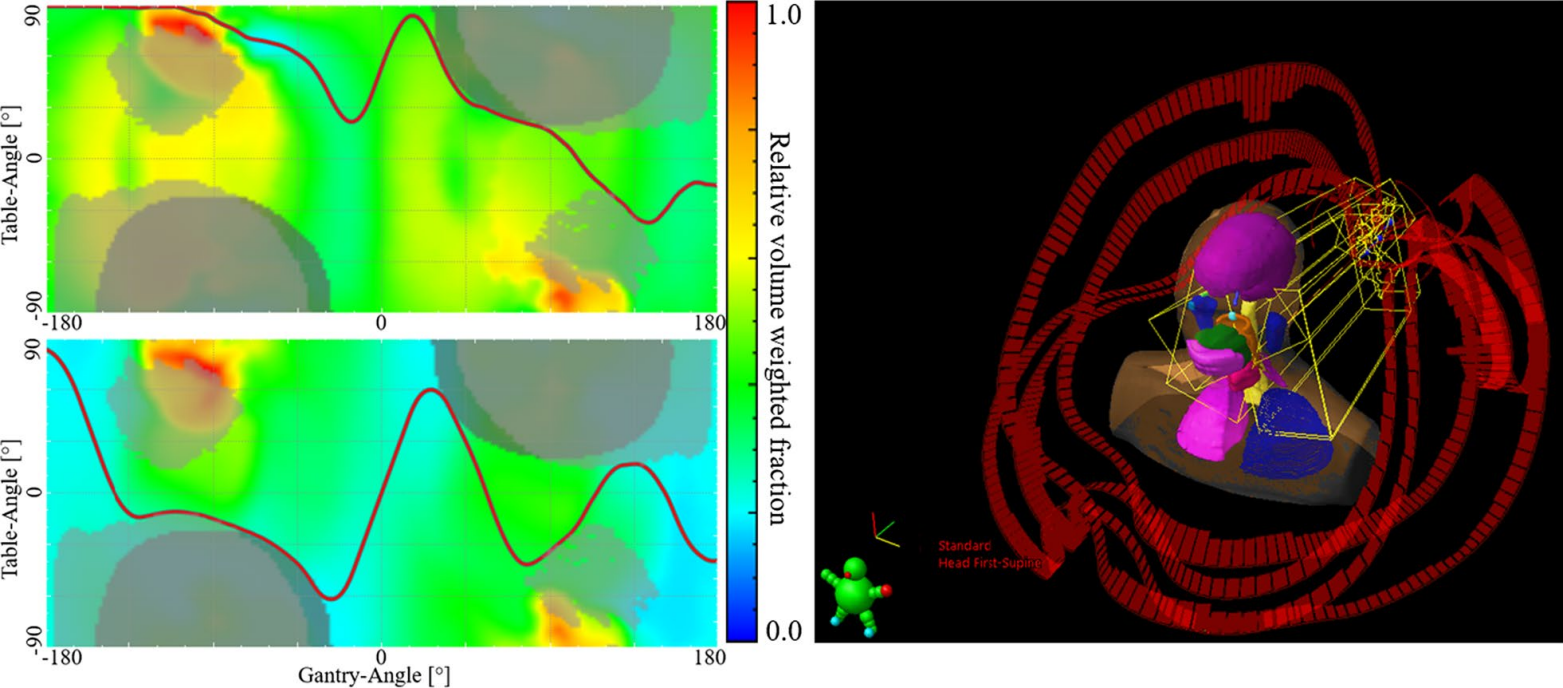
Example GT-maps with A*-determined paths (red curve) in the path-finder framework (left) and imported into Eclipse for the 50 Gy dose level of HN2. Light grey areas indicate collision zones, dark grey areas indicate end-of-CT restrictions. OAR selection for each map is detailed in Table 1. Individual OAR maps are shown in Additional file 1: Fig. 1
